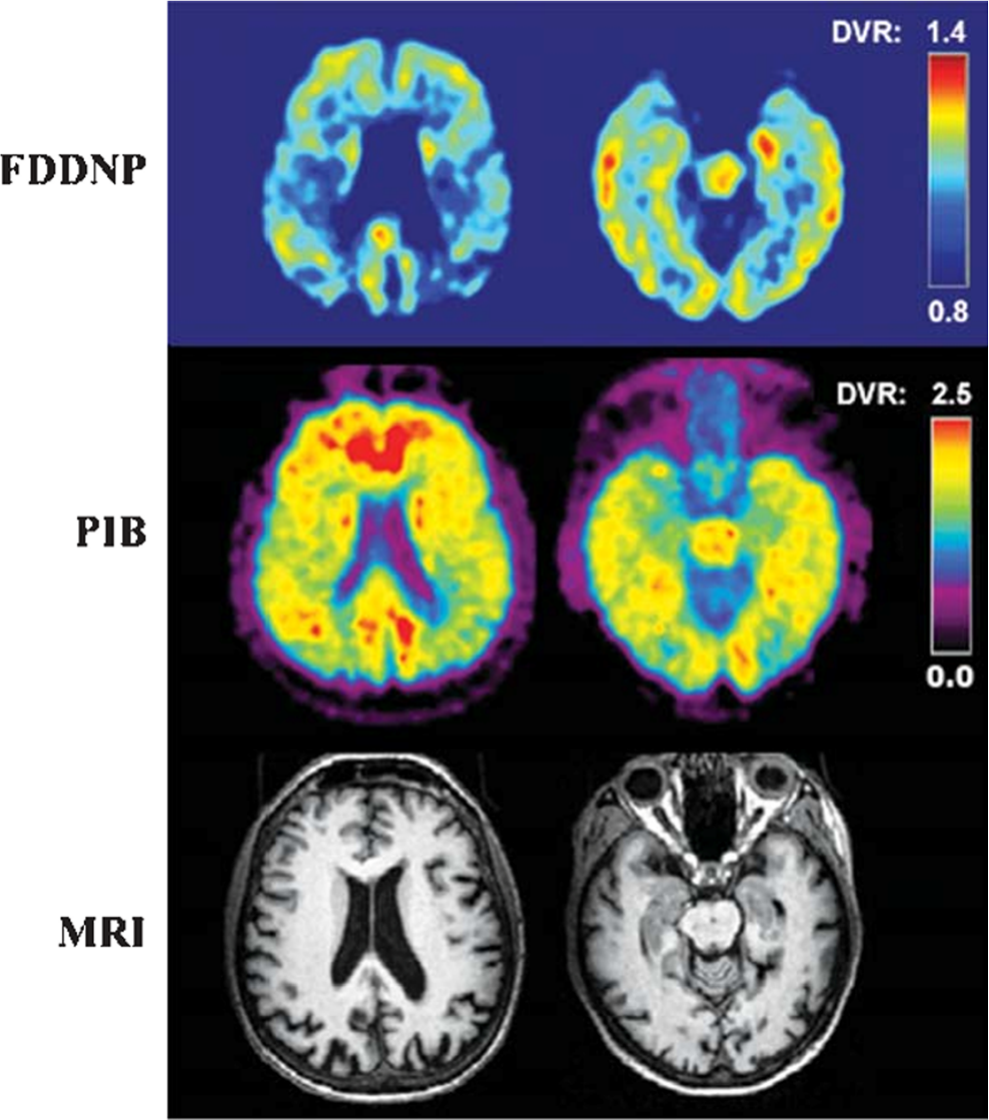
[^18^F]FDDNP-PET images. Co-registered FDDNP-PET, PIB-PET and MRI scans of a patient with Alzheimer’s disease. Images in the left column: parietal lobe; images in the right column: temporal lobe. [16]. (Reprinted from J. Alzheimers Dis;26. Shin J, Kepe V, Barrio JR, Small GW. The merits of FDDNP-PET imaging in Alzheimer’s disease., Suppl 3:135–145. Copyright (2011), with permission from IOS Press”.) The publication is available at IOS Press through 10.3233/JAD-2011-0008
